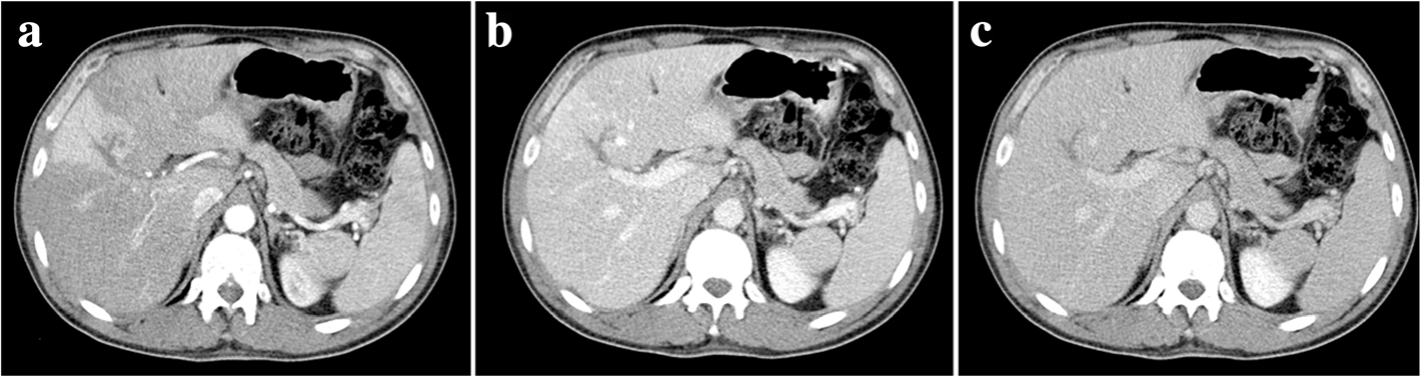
Portal vein thrombosis with THED. a Arterial phase CT axial image shows a triangular-shaped area of arterial enhancement, corresponding to the parenchyma formerly supplied by an occluded right anterior PV branch. At portal venous (b) and late phases (c) the hepatic parenchyma homogenizes, and there is persistent absence of venous enhancement due to bland thrombus
